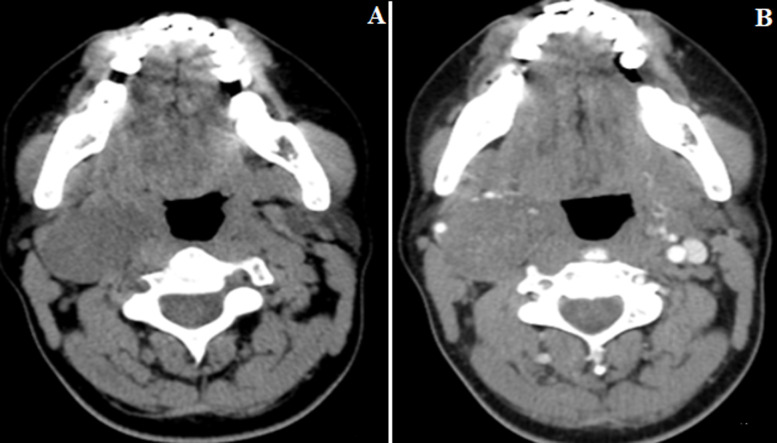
non-contrast (A) and contrast-enhanced (B) CT images; the mass was located lateral to the right submandibular region contrast-enhanced CT demonstrated enhancement of intratumoral blood vessels
